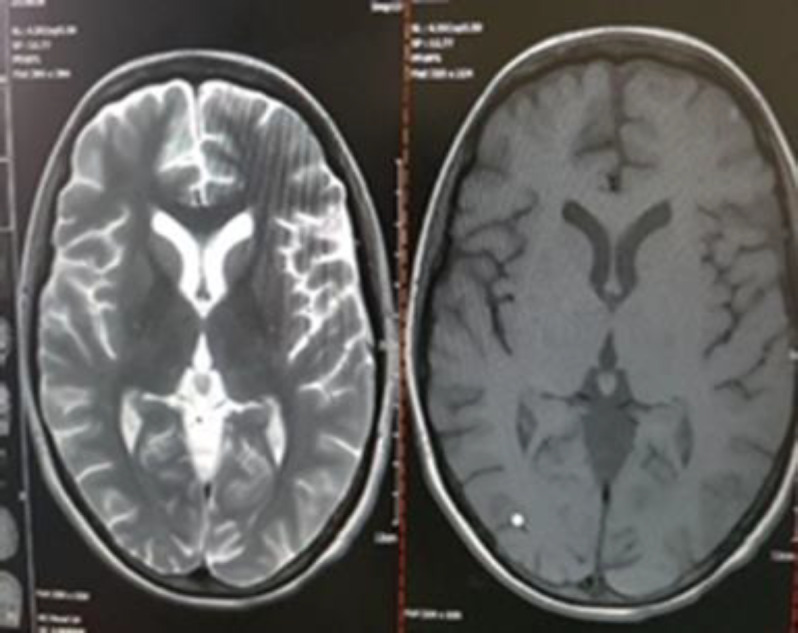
Follow-up MRI after three weeks of combination therapy and four sinus debridement procedures
